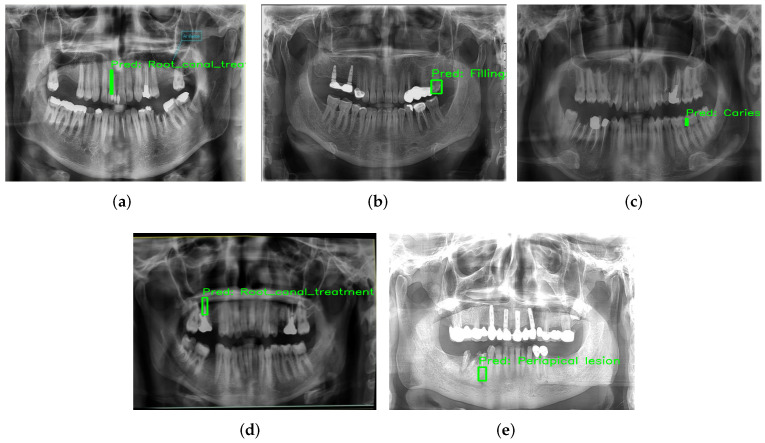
Representative qualitative results from the InceptionV3 model, showcasing the successful classification of various dental conditions. (a) Root canal treatment, (b) Filling, (c) Caries, (d) Root canal treatment and (e) Periapical lesion.
